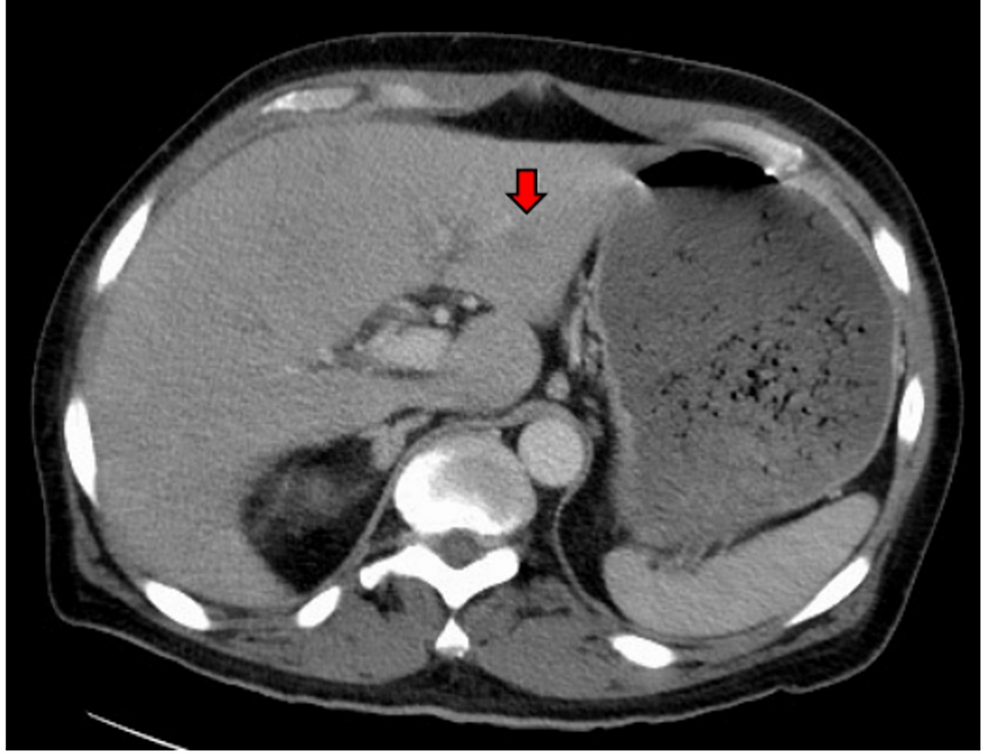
CT abdomen (axial view) showing small ill-defined hypoattenuating area within the left lobe of the liver in segment III measuring 12 x 13 x 15 millimeters (shown with a red arrow). CT: computed tomography.
